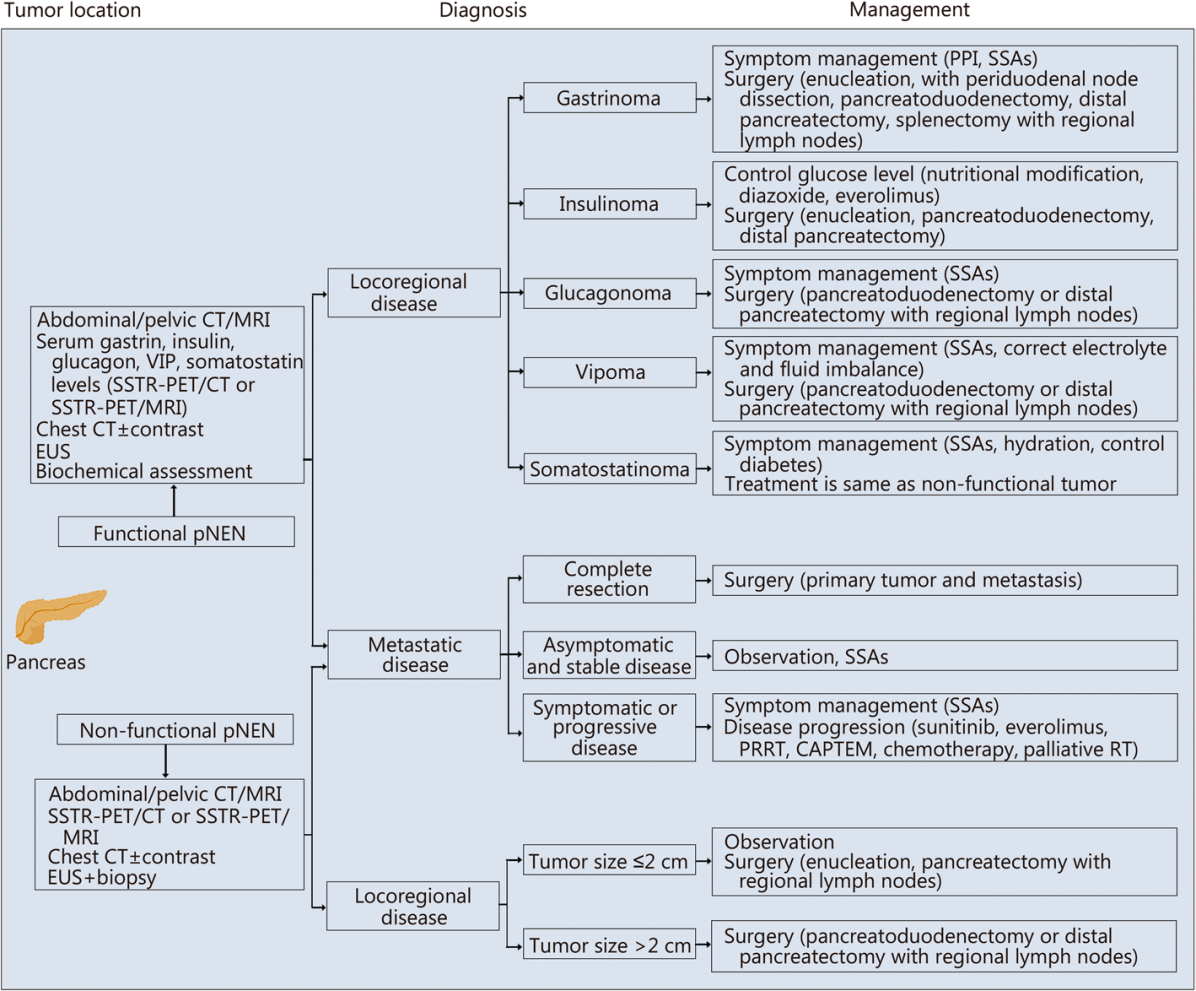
The diagnosis and management of pNENs. The management of functional and non-functional pNENs differs. However, similar examinations, including MRI, chest CT, and biopsy, can be performed for the diagnosis of these tumors. Disease management depends on the disease type, tumor size, and functional or non-functional status. EUS endoscopic ultrasound, US ultrasound, CT computed tomography, MRI magnetic resonance imaging, SSTR-PET/CT somatostatin receptor-positron emission tomography/computed tomography, PPI proton pump inhibitor, LAR long-acting release, pNEN pancreatic neuroendocrine neoplasm, SSAs somatostatin analogs, RT radiotherapy, PRRT peptide receptor radionuclide therapy, CAPTEM capecitabine and temozolomide, VIP vasoactive intestinal polypeptide
